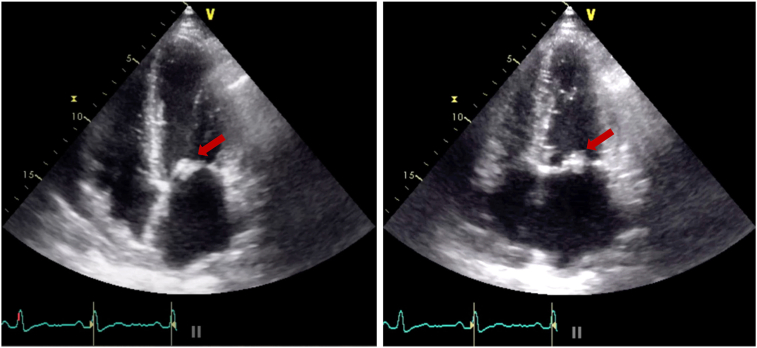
Two-dimensional TTE, apical 4-chamber diastolic (*left*) and systolic (*right*) views, demonstrates normal left ventricular size and function and a large echogenic mass (*arrows*) attached to the PMVL causing restricted leaflet mobility.
